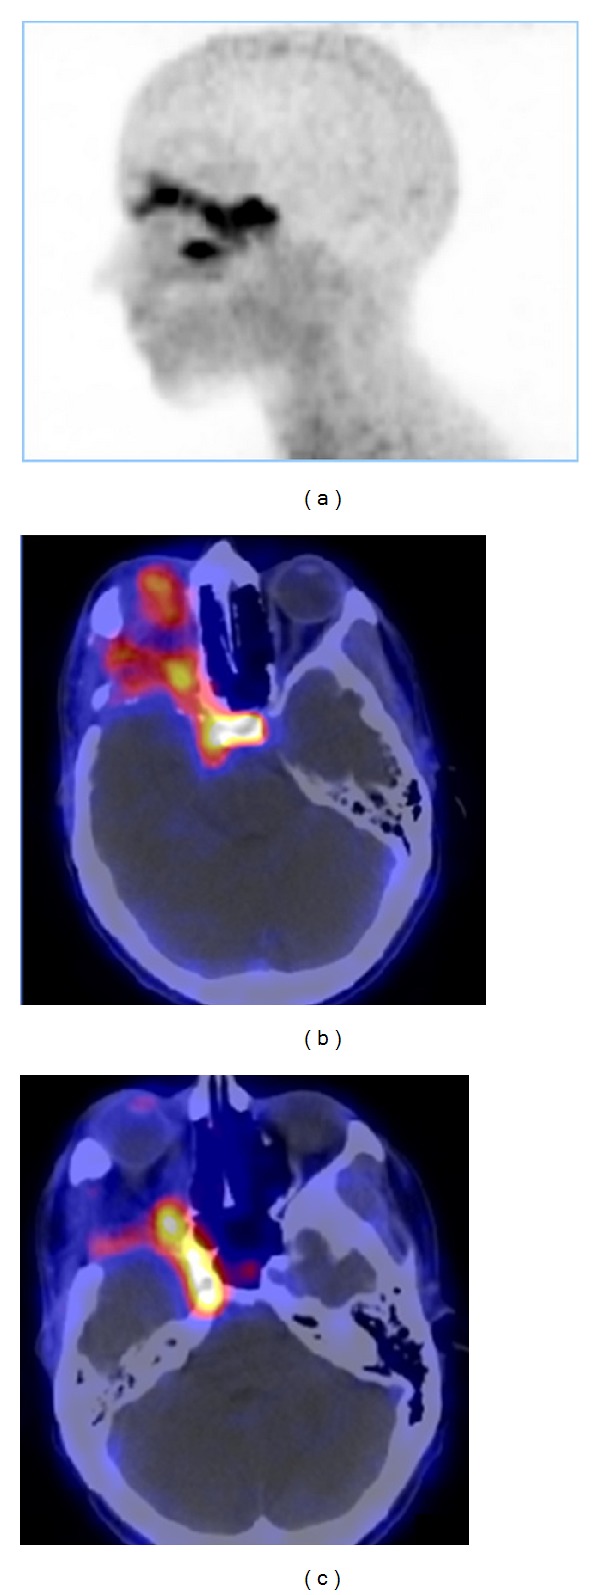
^68^Ga-DOTATOC PET (a) and fused PET/CT (b and c) images of a skull base meningioma with orbital invasion and close relation to the sella turcica region. Physiological tracer uptake of the pituitary gland [106].
